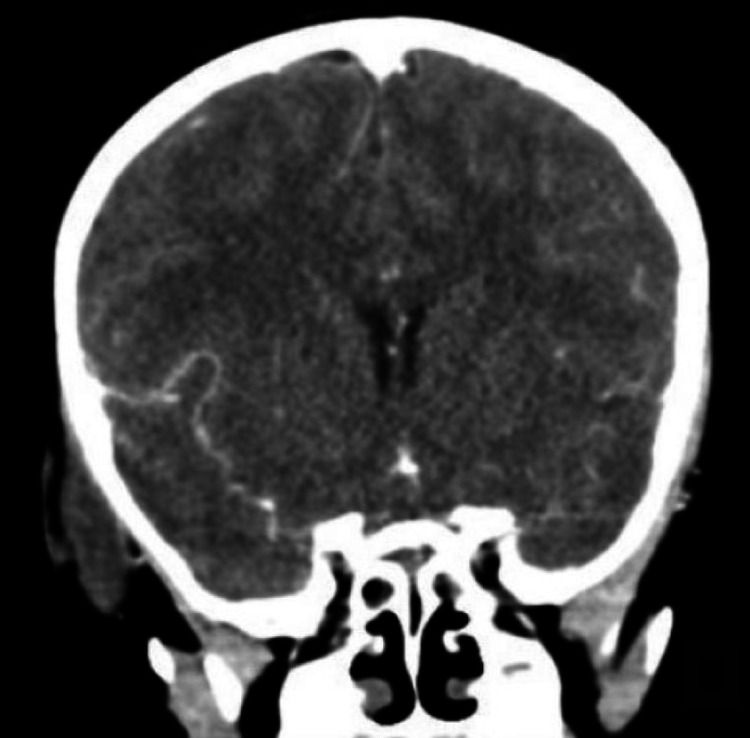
A preoperative contrast computed tomography scan showing a hypodense mass (approximately 5 cm in diameter) involving the right temple, extending to the superior parotid gland, and sparing the right external auditory canal
